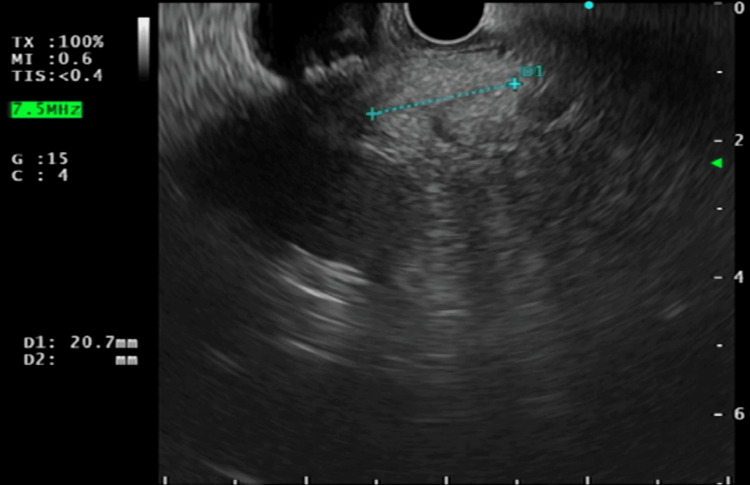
Endoscopic ultrasound image showing a peri-pancreatic lymph node (20.7 mm) in cross section
